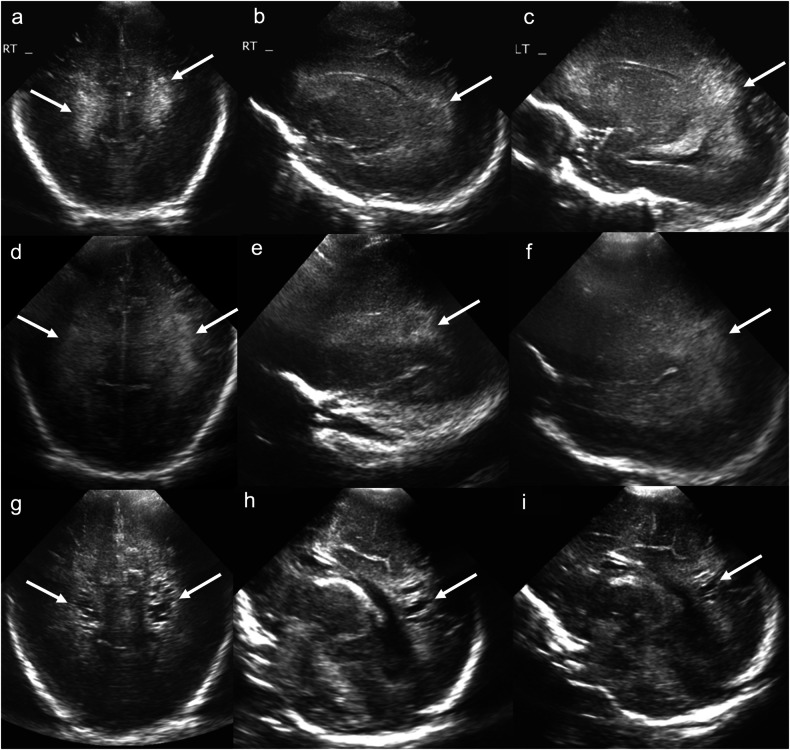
Periventricular leukomalacia. Coronal images acquired with a medium-frequency (3–10 mHz) curved probe at the level of the parietal and occipital lobes (a, d, g) and right (b, e, h) and left (c, f, i) parasagittal images acquired with the same probe at the level of the lateral ventricles on days 1 (a–c), 14 (d–f) and 28 (g–i) of life in a 31-week preterm neonate. a–c Note heterogenous increased echogenicity of the posterior periventricular white matter bilaterally (solid arrows). d–f This heterogenous white matter increased echogenicity (left greater than right) persisted on the follow-up ultrasound 2 weeks later. g–i At day 28, there has been interval development of bilateral occipital and posterior periventricular cysts with persistent increased echogenicity of the surrounding white matter consistent with cystic PVL
